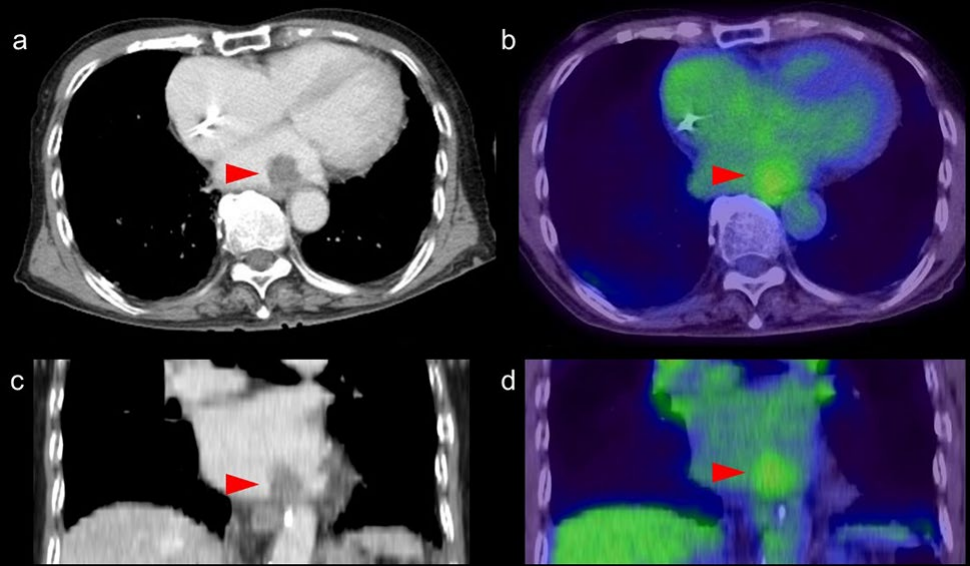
Myxoma arisen in left atrium. Contrast enhanced CT imaging demonstrated mass lesion in left atrium, which showed low FDG uptake
